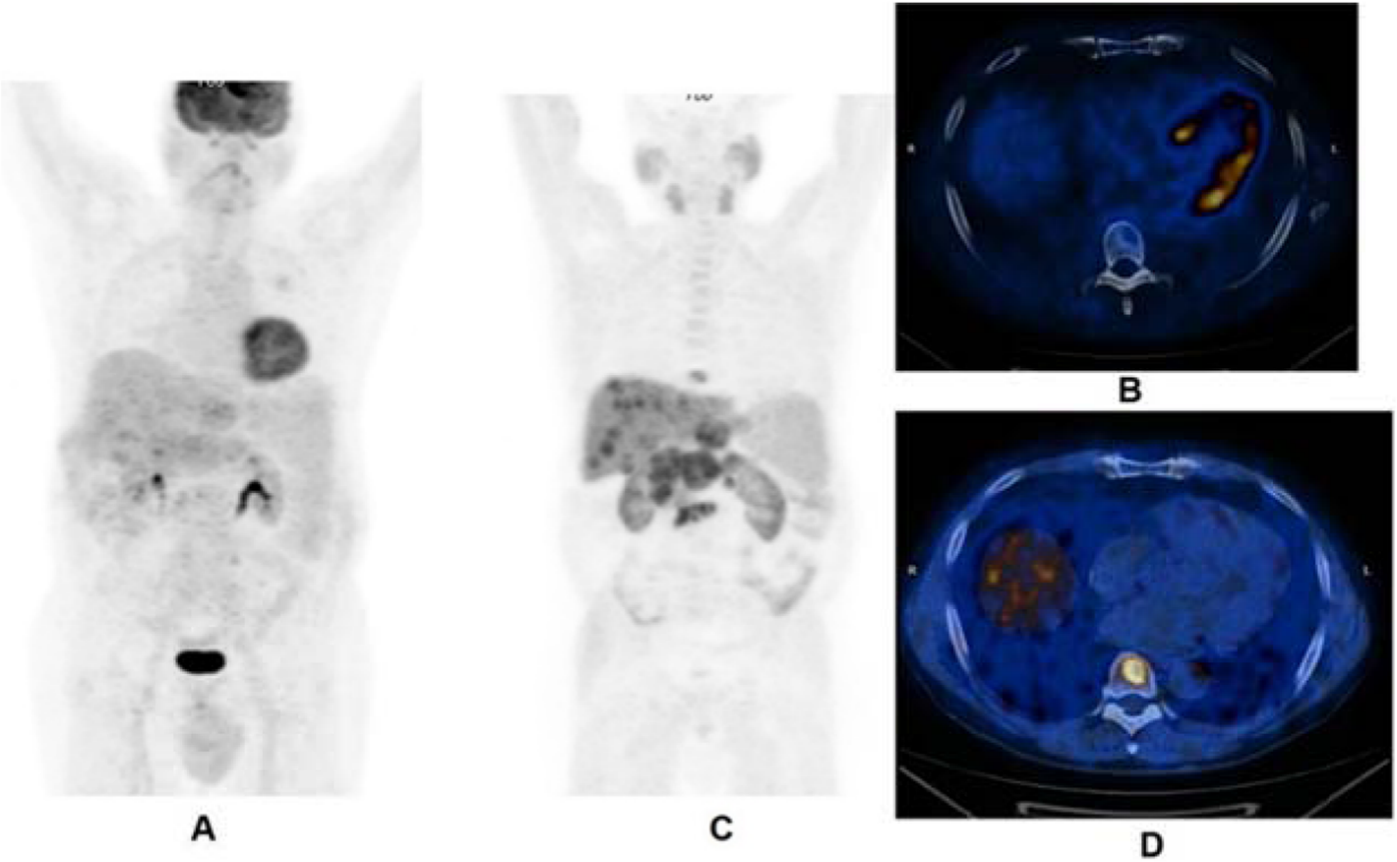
^11^C-acetate, staging hepatocellular carcinoma (HCC), comparison with 18F-FDG. *Clinical history*: 78 y.o. man with metastatic HCC after liver transplantation, patient underwent acetate and FDG study in a single day examination. *PET/CT findings*: acetate: multiple areas of increased uptake in the liver, lymph nodes, and bones consistent with lytic lesions at CT images. No FDG uptake. a FDG MIP. b FDG fused images. c acetate MIP. d acetate fused images
